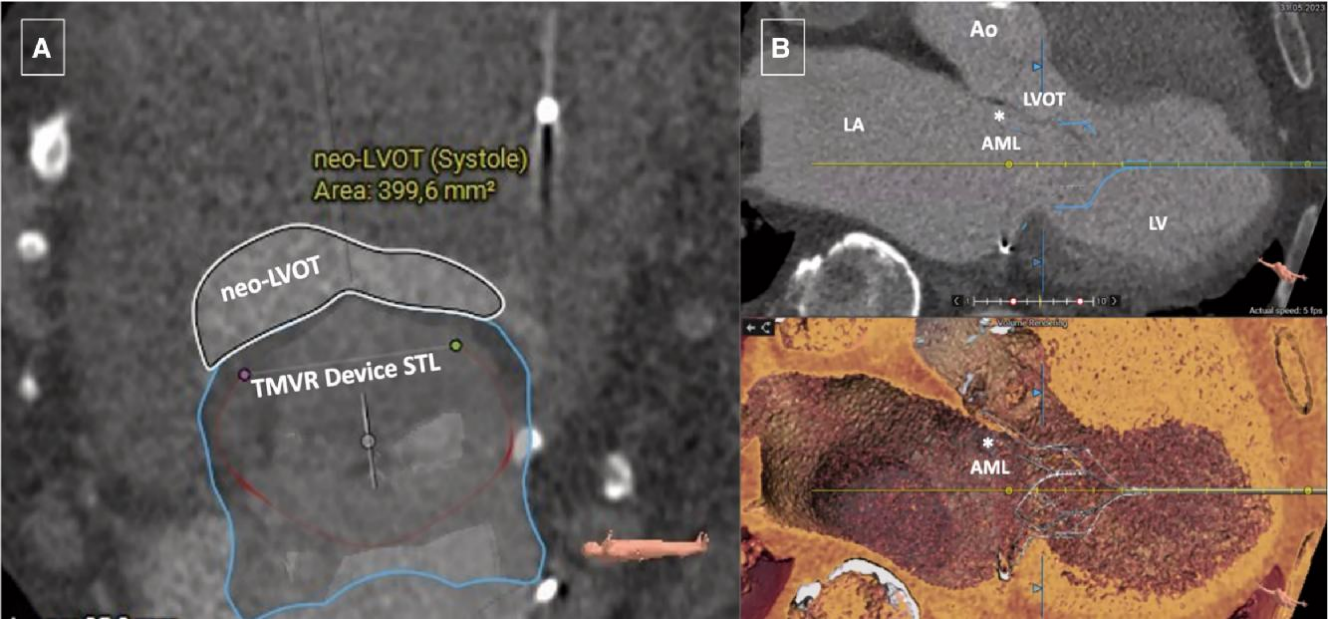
Pre-procedural cardiac CT assessment, following the deployment of a stereolithographic file of a SP 33M Tendyne system (Abbott Structural Heart, Santa Clara, CA, USA), highlights the risk of dynamic LVOT obstruction and SAM. (*A*) Predicted neo-left ventricular outflow tract (neo-LVOT) area after virtual device implantation at end-systole. (*B*) Elongated AML (asterisk). Ao, aorta; LA, left atrium; LV, left ventricle; STL, stereolithography; TMVR, transcatheter mitral valve replacement; CT, computed tomography; LVOT, left ventricle outflow tract; SP, standard profile; SAM, systolic anterior motion of the mitral valve; AML, anterior mitral valve leaflet.
